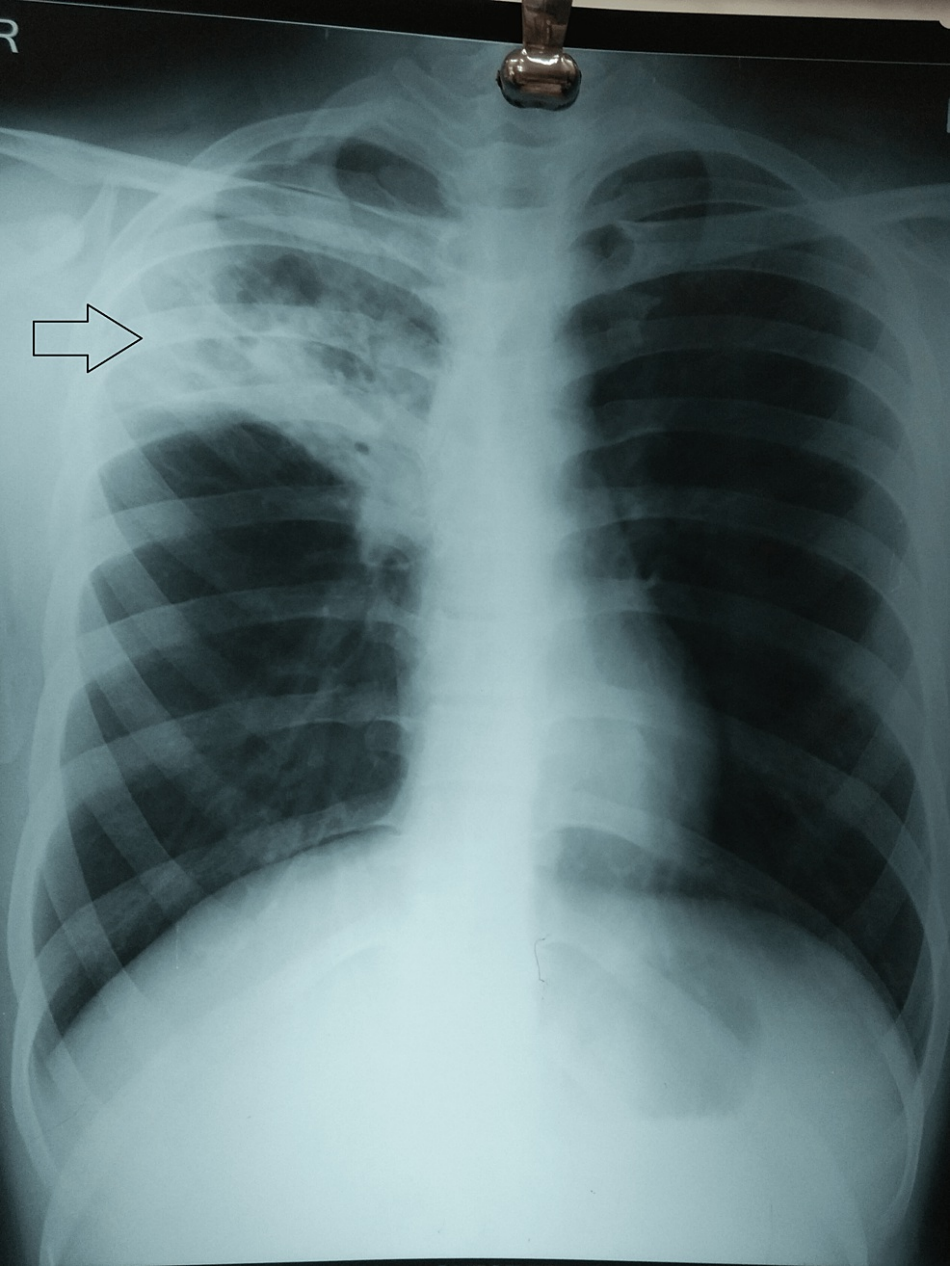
Chest radiograph posteroanterior (PA) view suggestive of consolidations at the upper lobe of the right lung with indistinct borders.
